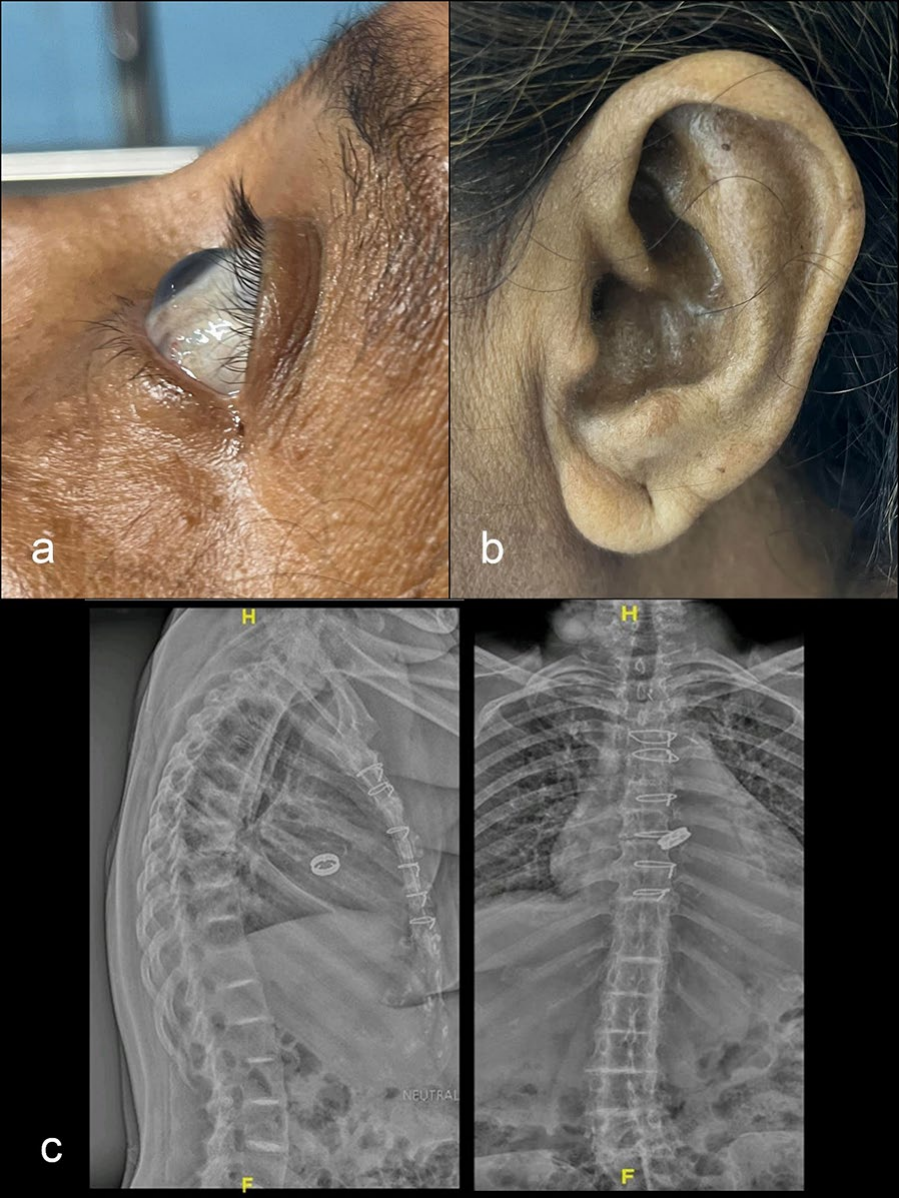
Characteristics of patients. a bluish-black discolouration of sclera of eye; b bluish-black discolouration of ear pinna; c dorsolumbar spine radiograph lateral and AP view showing intervertebral disc space calcifications
